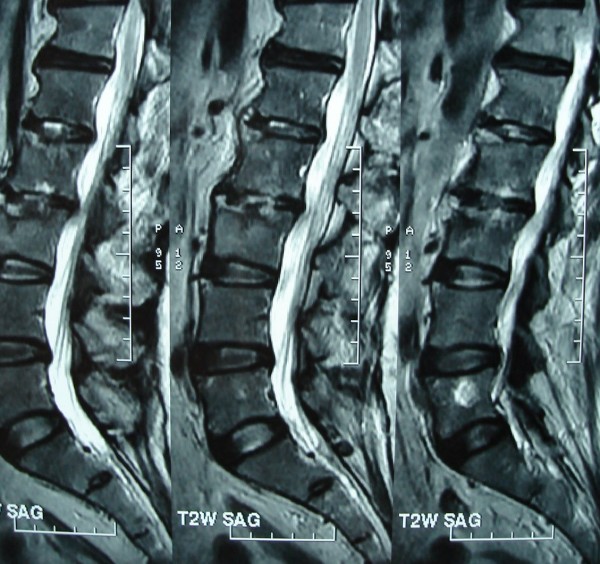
sagittal T1 MRI showing diskitis and vertebral signal changes suggestive of osteomyelitis
